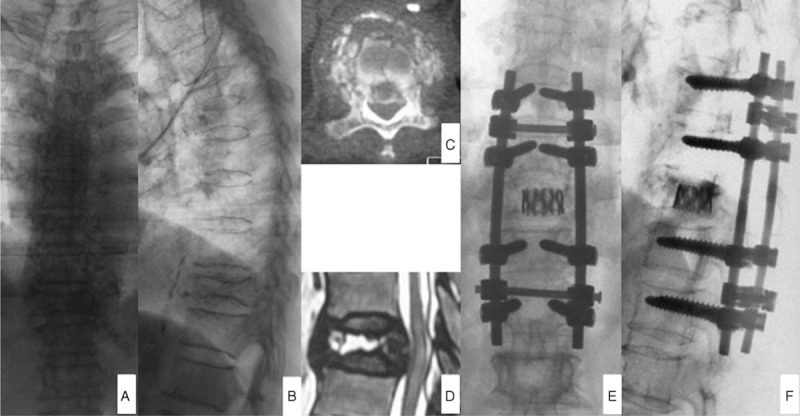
Female patient, 70-year old, was conducted posterior transpedicular internal fixation of titanium mesh supporting grafting bone after 2 months of chest and lower back pain accompanied by lower limbs activity disorder. Figures A and B represented the anteroposterior and lateral x-ray before operation, showing T10 fracture; figures C and D were MRI and CT taken before operation, showing T10 fracture and III stage of Kümmell's disease; and figures E and F were x-ray after anterior operation, manifesting the fine internal fixation position and satisfactory kyphosis correction.
